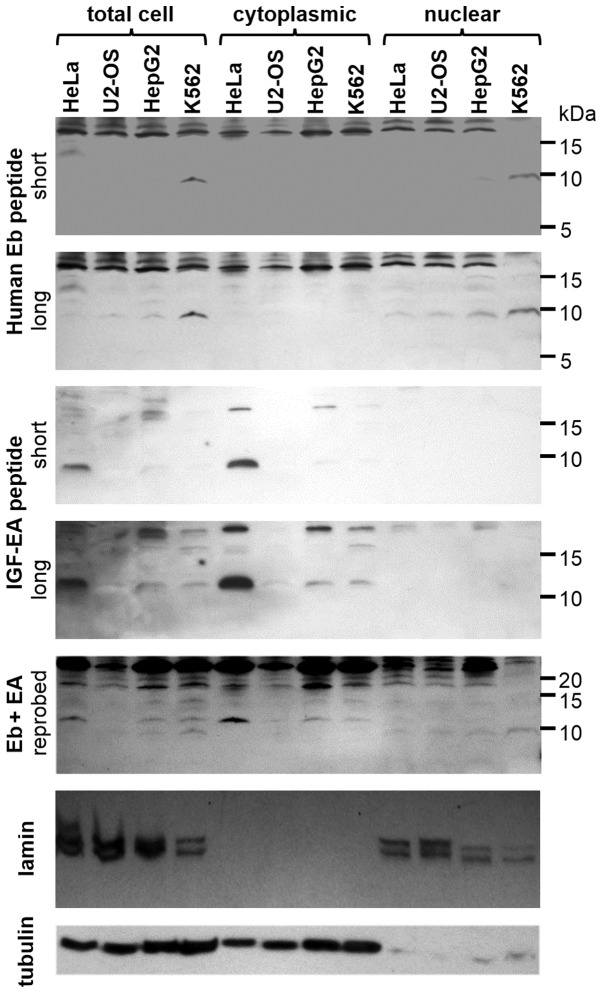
Immunoblotting showing endogenous IGF isoforms in human cancer cells. Sixty micrograms of protein were resolved in each lane. A 9 kD band is present in total cell extract and nuclear fraction from K562 cells and, to a smaller extent, in HepG2 when probed with anti-Eb peptide antibody on the first membrane (short exposure to X-ray film). After longer exposure, this band was also slightly visible in the other two cell lines used in this study (HeLa, U2OS), second panel. It never appeared in cytoplasmic fraction of these cells. A 11–12 kD band was detected when the same membrane was probed with anti-EA antibody, corresponding to human pro-IGF-1A (panel 3). However, this band was evident only in total cell extract and cytoplasmic fraction and never present in the nuclear one (panel 4). Also, another blotting is shown (panel 5), the same membrane already probed with anti-Eb antibody was stripped and reprobed with anti EA-peptide antibody. A merge of both anti-Eb and anti-EA pattern can be observed and a molecular mass difference of 2–3 kD between human Eb-peptide (9 kD) and pro-IGF-1A (11–12 kD). For confirmation of cellular fractionation, 2 different antigens are shown: lamin (nuclear marker), tubulin (cytoplasmic marker) (panels 6 and 7). IGF, insulin-like growth factor.
